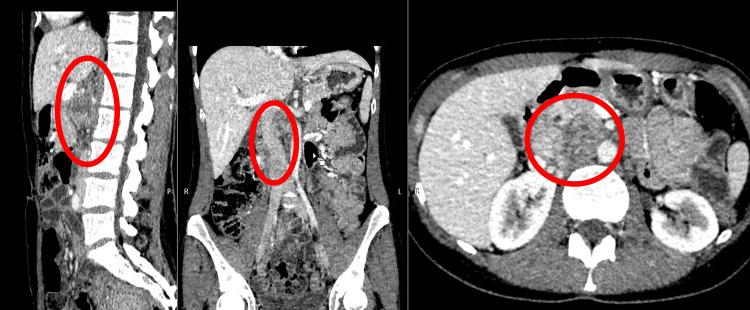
CT angiography of the aorta and its branches (2019), demonstrating the relationship between the tumor and major vascular structures.
